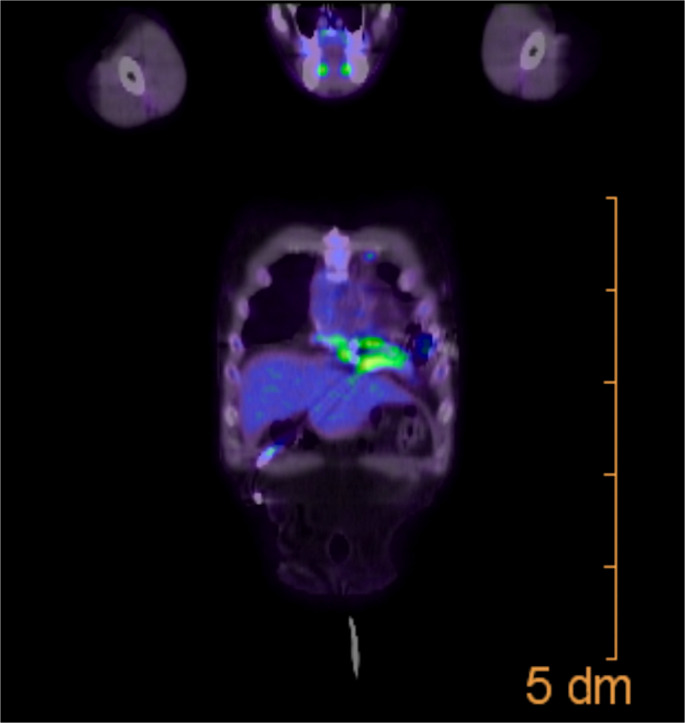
PET-CT scan indicating a multifocal endoplastitis of the LVAD including a hypermetabolic abscess in the upper mediastinum
